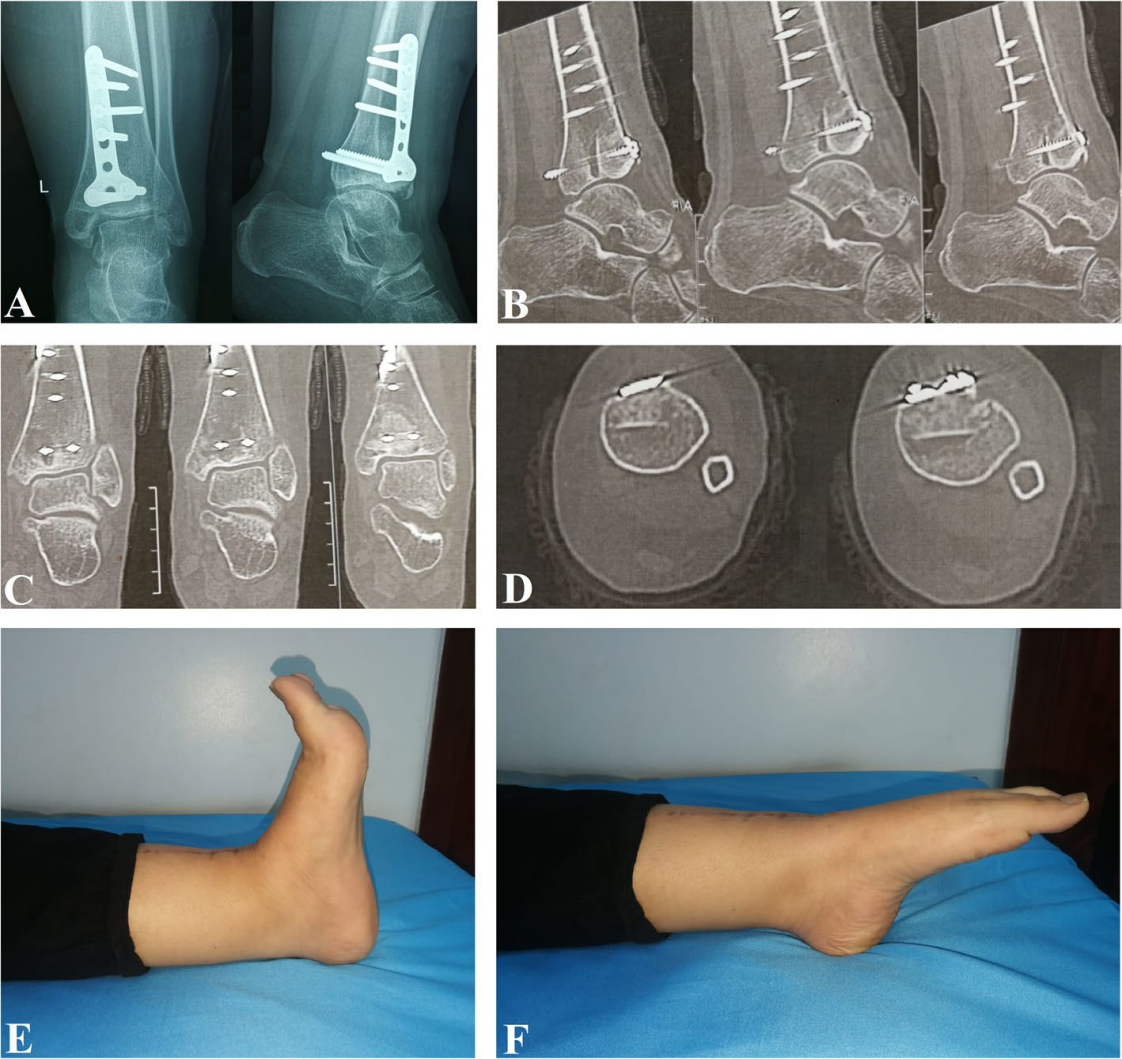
Six months follow-up radiological images showing complete union of the graft and reconstruction of the distal tibial articular surface. No donor site morbidity symptoms were reported. Clinical photos showing pain-free full range of motion of the ankle joint. A Anteroposterior and lateral plain X-rays. B Sagittal CT cuts. C Coronal CT cuts. D Axial CT cuts. E Ankle dorsiflexion. F Ankle plantar flexion
